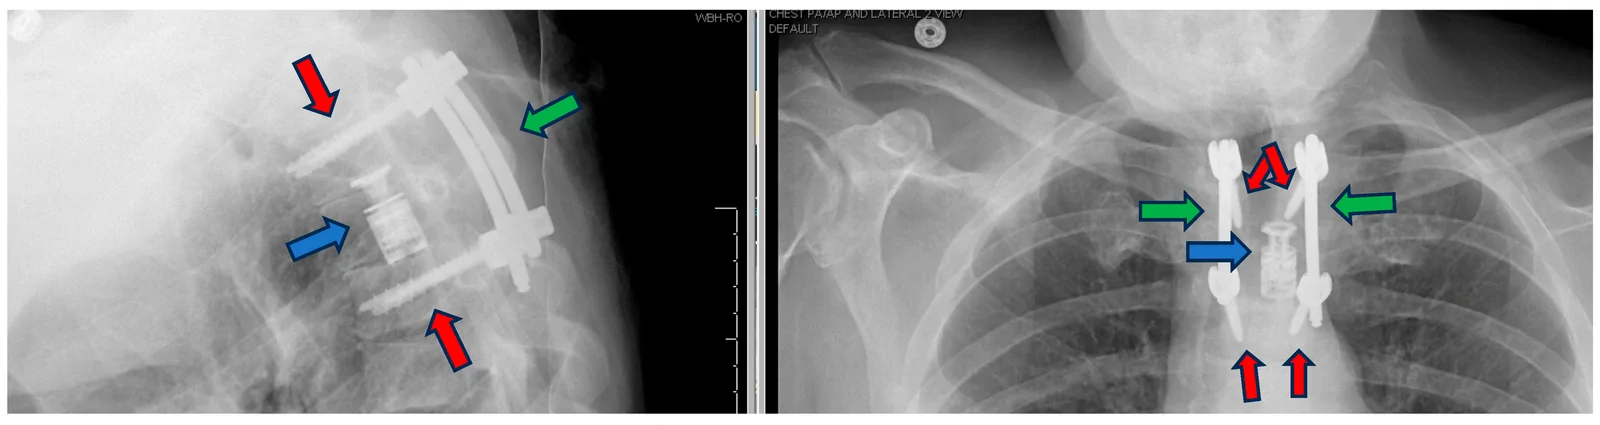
Postoperative lateral X-ray showing expandable cage vertebral body reconstruction with short-segment instrumentation from T4 to T6. The expandable cage is denoted by a blue arrow. The screws are denoted by red arrows. The rods are denoted by green arrows.
